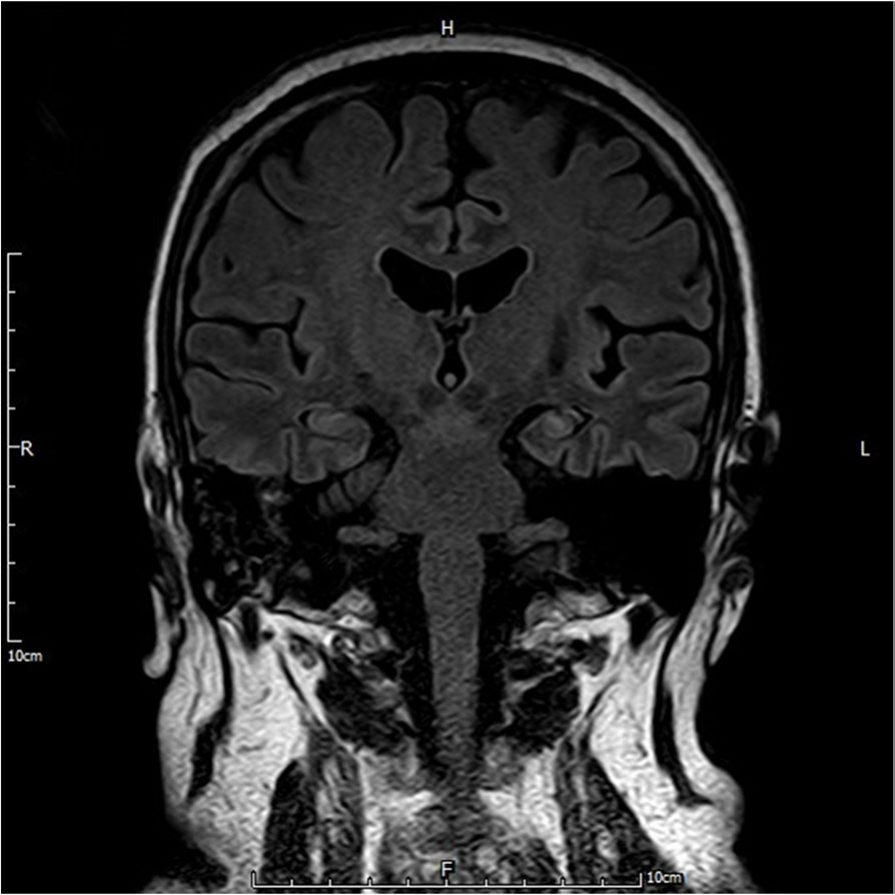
MRI sequence at 5-month follow up showed the left hippocampus was smaller than the contralateral side
